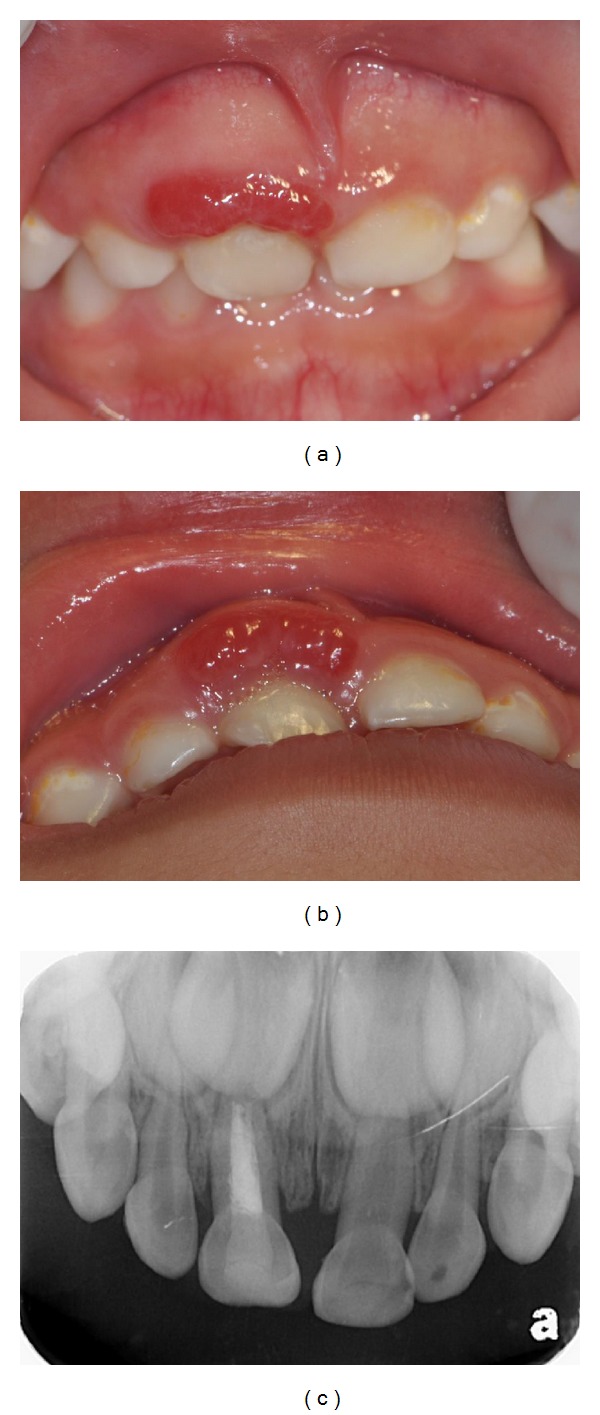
Clinical and radiographic appearances. (a) Frontal view: a fiery red gingival lesion on the labial side of the primary maxillary right central incisor. (b) Occlusal view: the maxillary right primary central incisor was a little palatally displaced. (c) A pulpectomy and restoration had been done for the primary maxillary right central incisor at a local dental clinic. There was no observable radiopaque abnormality in the periapical radiograph.
